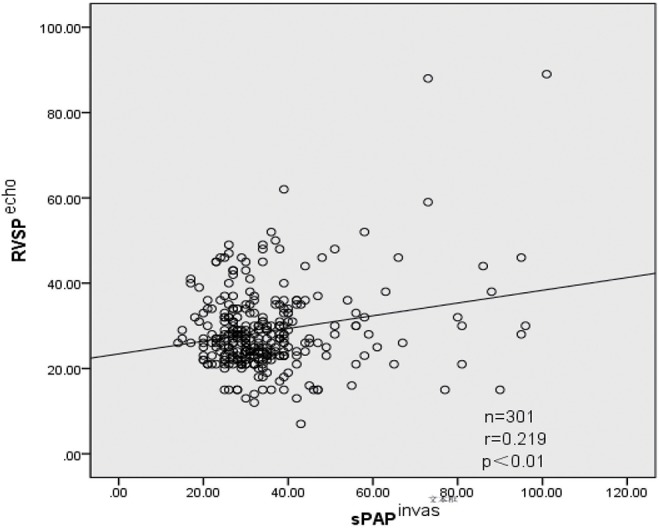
Relationship between RVSP estimated by D-ECHO and sPAP observed by RHC (*r* = 0.219, *p* < 0.01). Echo, measured by Doppler echocardiographic; invas, invasive measurement by right-heart catheterization; r, correlation coefficient (Pearson); sPAP, systolic pulmonary artery pressure; RVSP, right ventricular systolic pressure.
